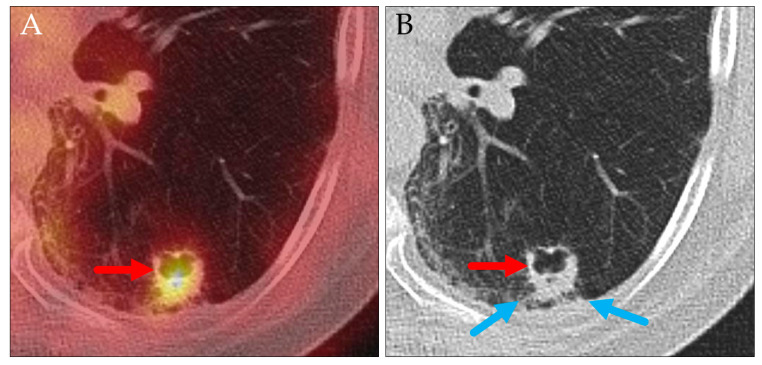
Axial PET/CT (A) and CT (B) of a patient with squamous cell carcinoma. Despite the small spiculae reaching the visceral pleura (blue arrows), the partially necrotic primary tumor (red arrows) was classified as cT1b. At the time of surgery more than three months later, a broad invasion of the visceral pleura was found (pT2a).
